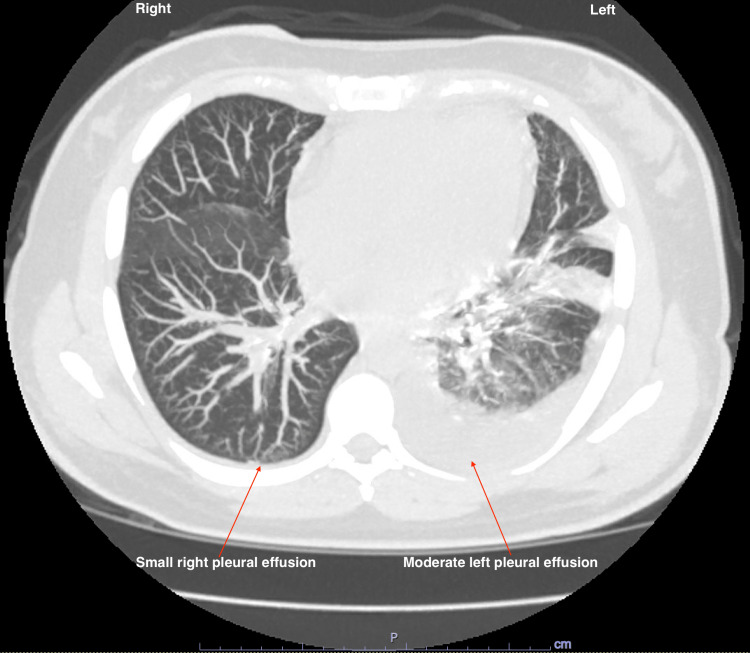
CT chest showing small right and moderate left pleural effusions
